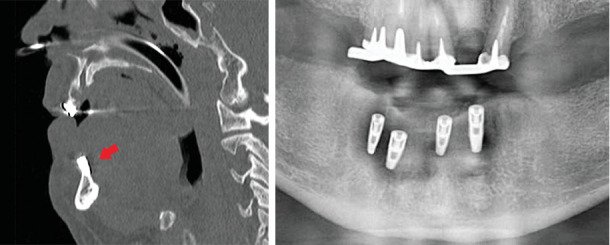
CT scan sagittal view. Nasotracheal tube with mouth floor and tongue displacement. The red arrow shows dehiscence at the lingual aspect of the fixtures. The OPT shows four implants incorrectly placed in the atrophic alveolar ridge.
